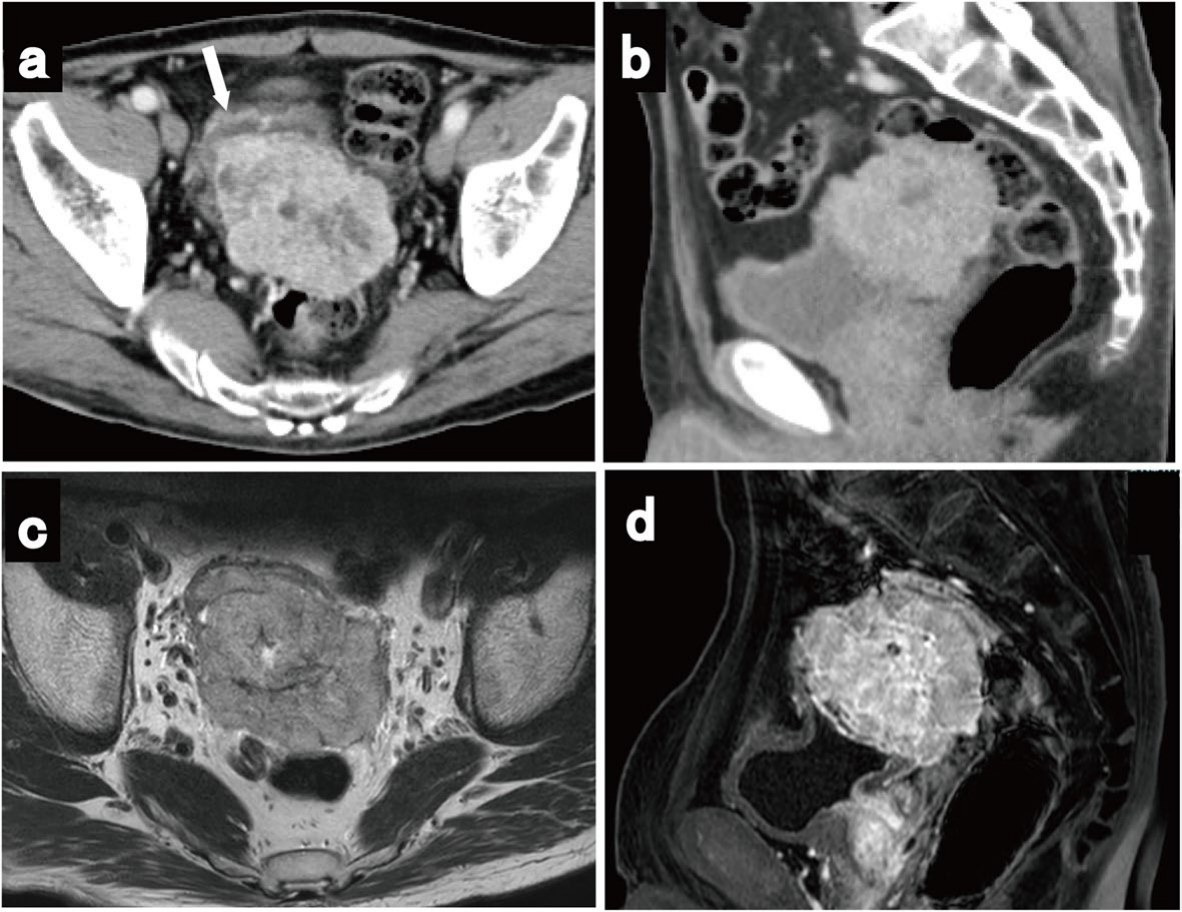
Contrast-enhanced computed tomography (CT), magnetic resonance imaging (MRI), and positron emission tomography/computed tomography findings. a Computed tomography (CT) revealing a tumor measuring 77 mm in diameter in the posterior wall of the urinary bladder (white arrow: urinary bladder), with heterogeneous enhancement in the solid part and an enhancing hypodense lesion. b Sagittal CT showing the tumor located between the posterior wall of the bladder and rectum. c MRI revealing a large tumor between the posterior wall of the bladder and rectum; the tumor is heterogeneous and partly hyperintense on a T2-weighted image. d Sagittal contrast-enhanced MRI showing an indistinct border between the tumor and posterior wall of the bladder, with the rectum compressed dorsally
